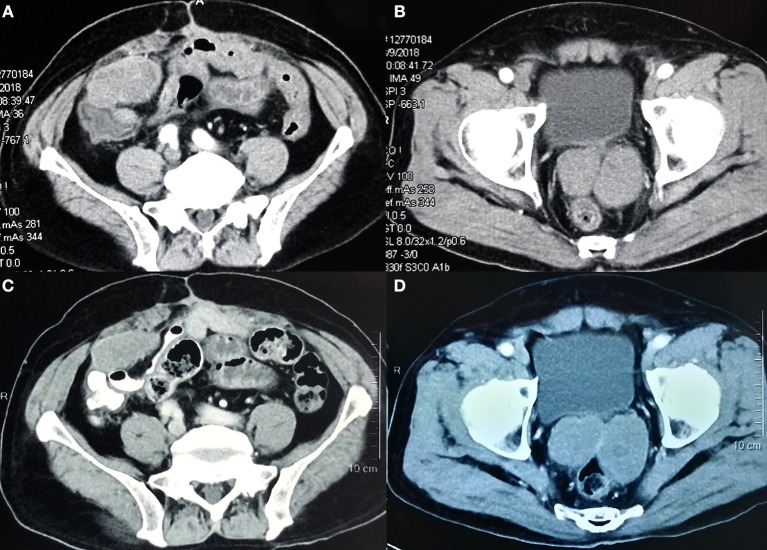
Abdominal computed tomography scans showing the metastatic lesions in the abdomen and pelvic cavity before treatment (A,B) to a stable disease after 8 months of treatment with apatinib (C,D).
